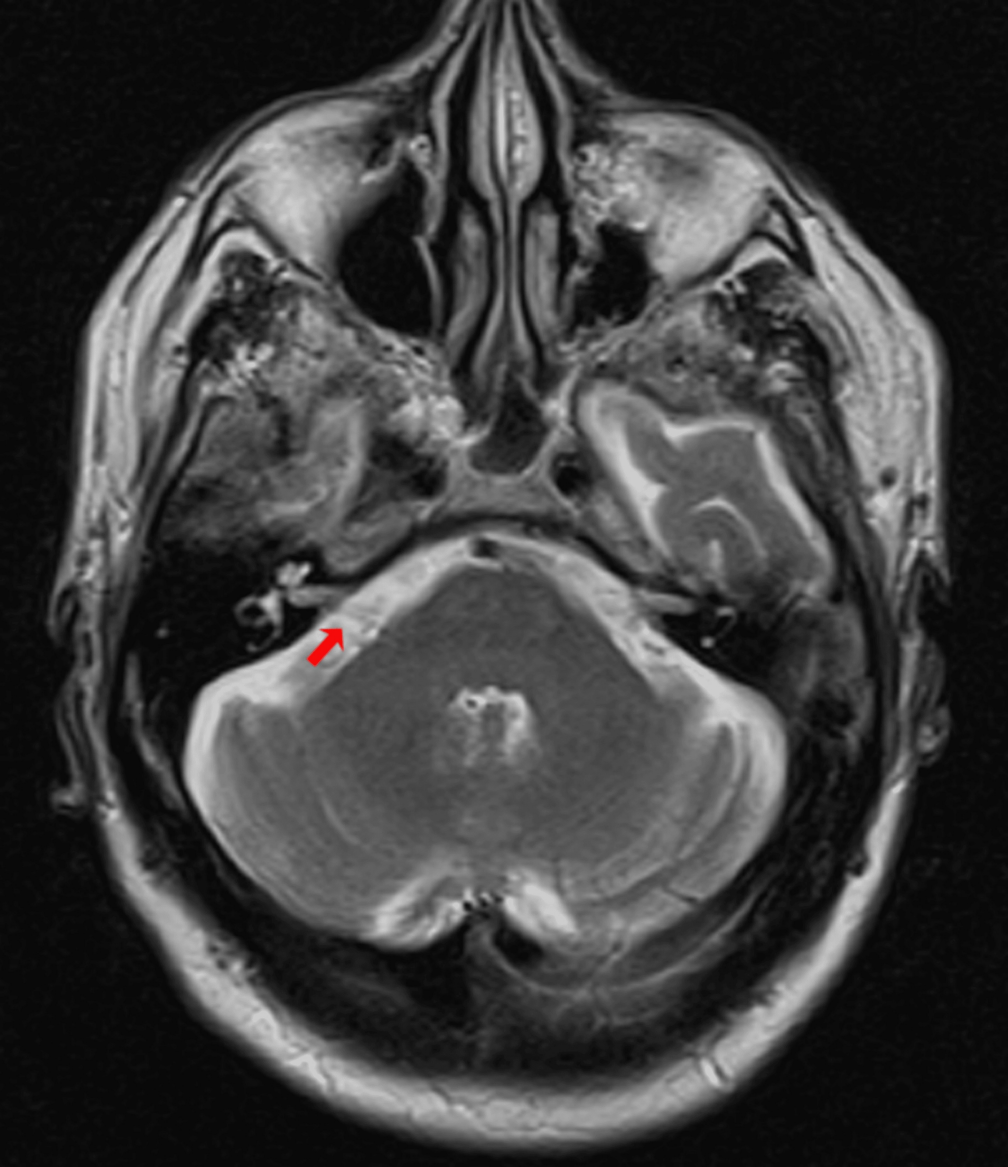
Axial T2-weighted brain MRI of patient no.3 Red arrow: Right-sided neurovascular compression (Chavda classification grade I)
